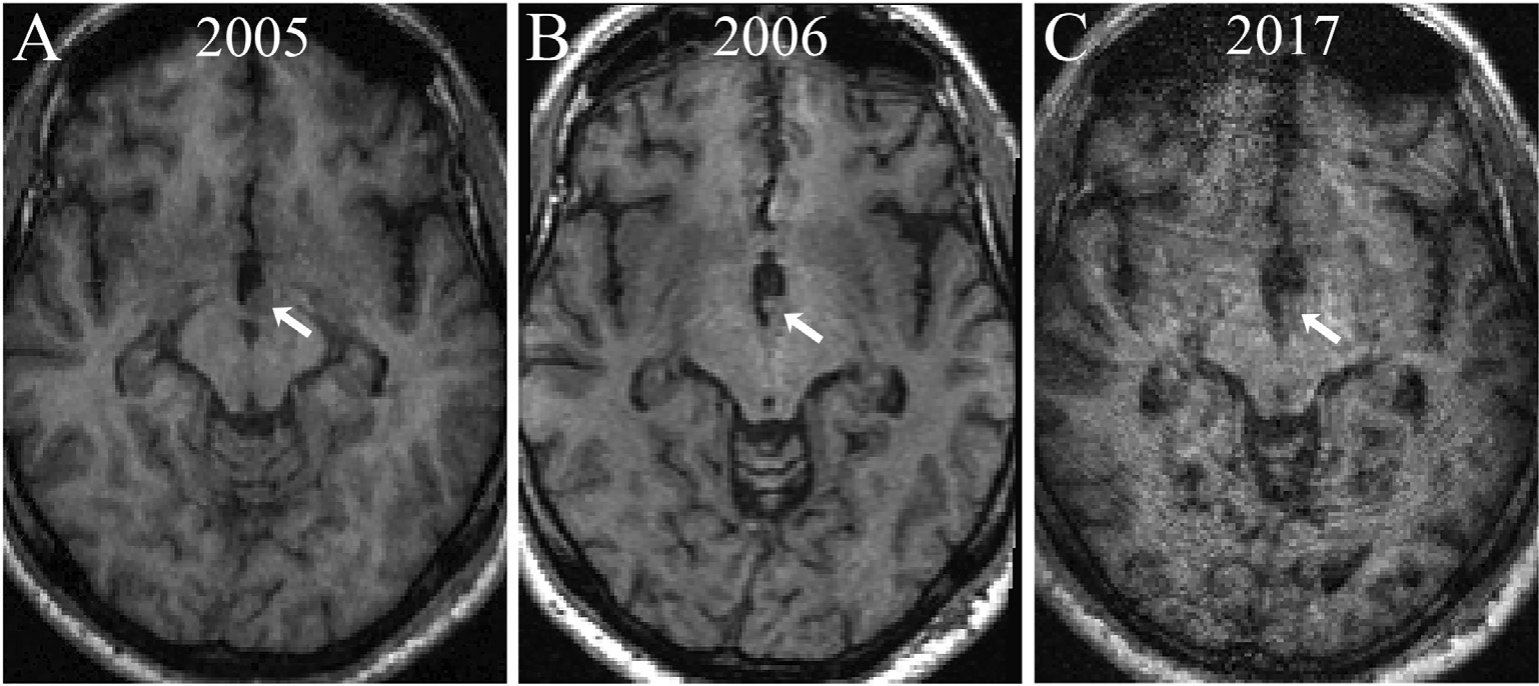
Three anatomic T1-weighted MRI axial images: During presurgical evaluation in 2005 (A) postsurgical evaluation in 2006 (B) and 2017 (C). Note that following initial surgical resection in 2006, the tumor volume was reduced to less than half of the original one. The arrow points to the intraventricular component of the tumor, in relation to the lateral inferior wall of the third ventricle.
